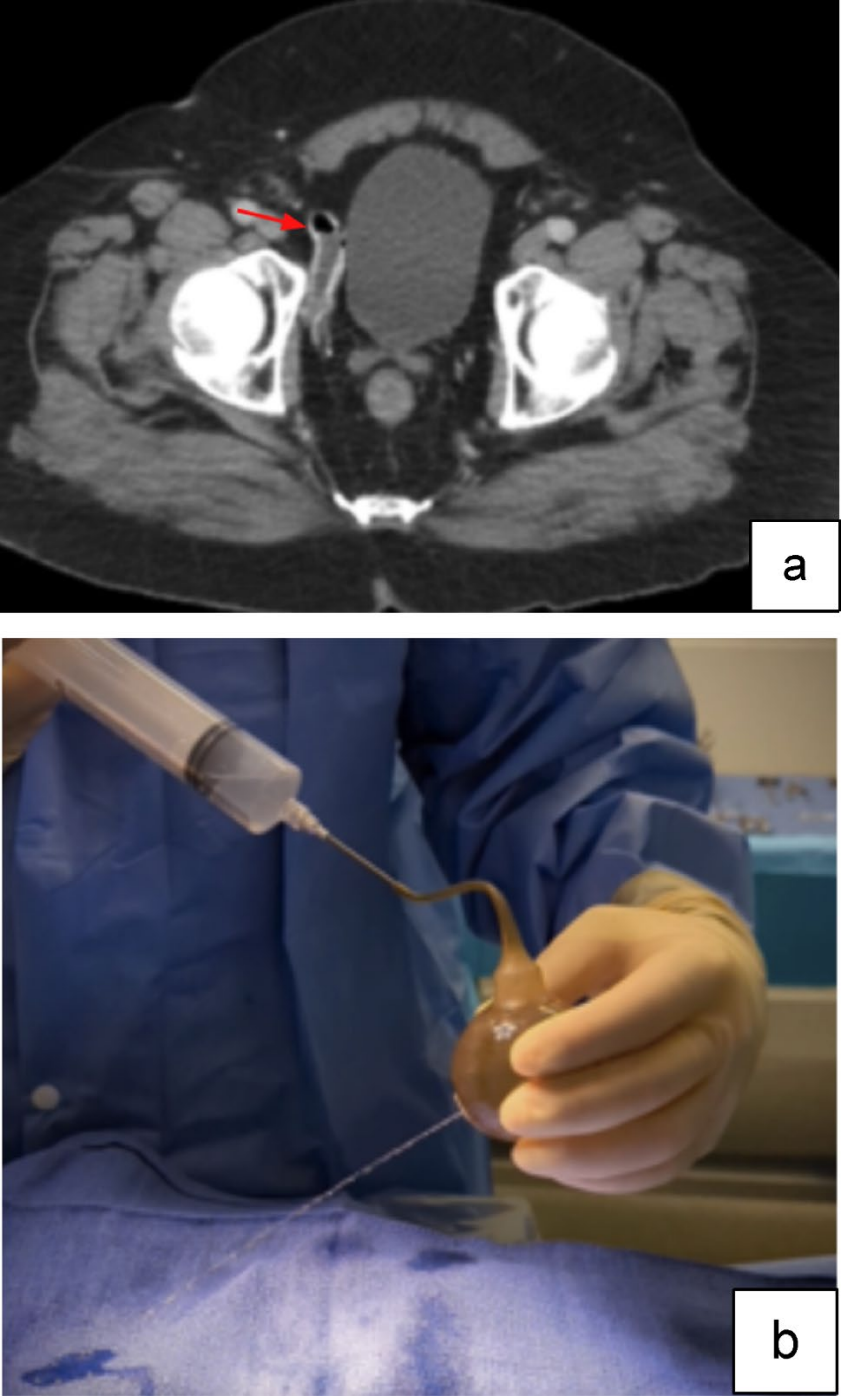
Axial CT image with IV contrast (a) shows a collapsed reservoir with an air-fluid level, compatible with system leak (red arrow). Intraoperative photo demonstrates pinpoint fluid leak from reservoir (b)
